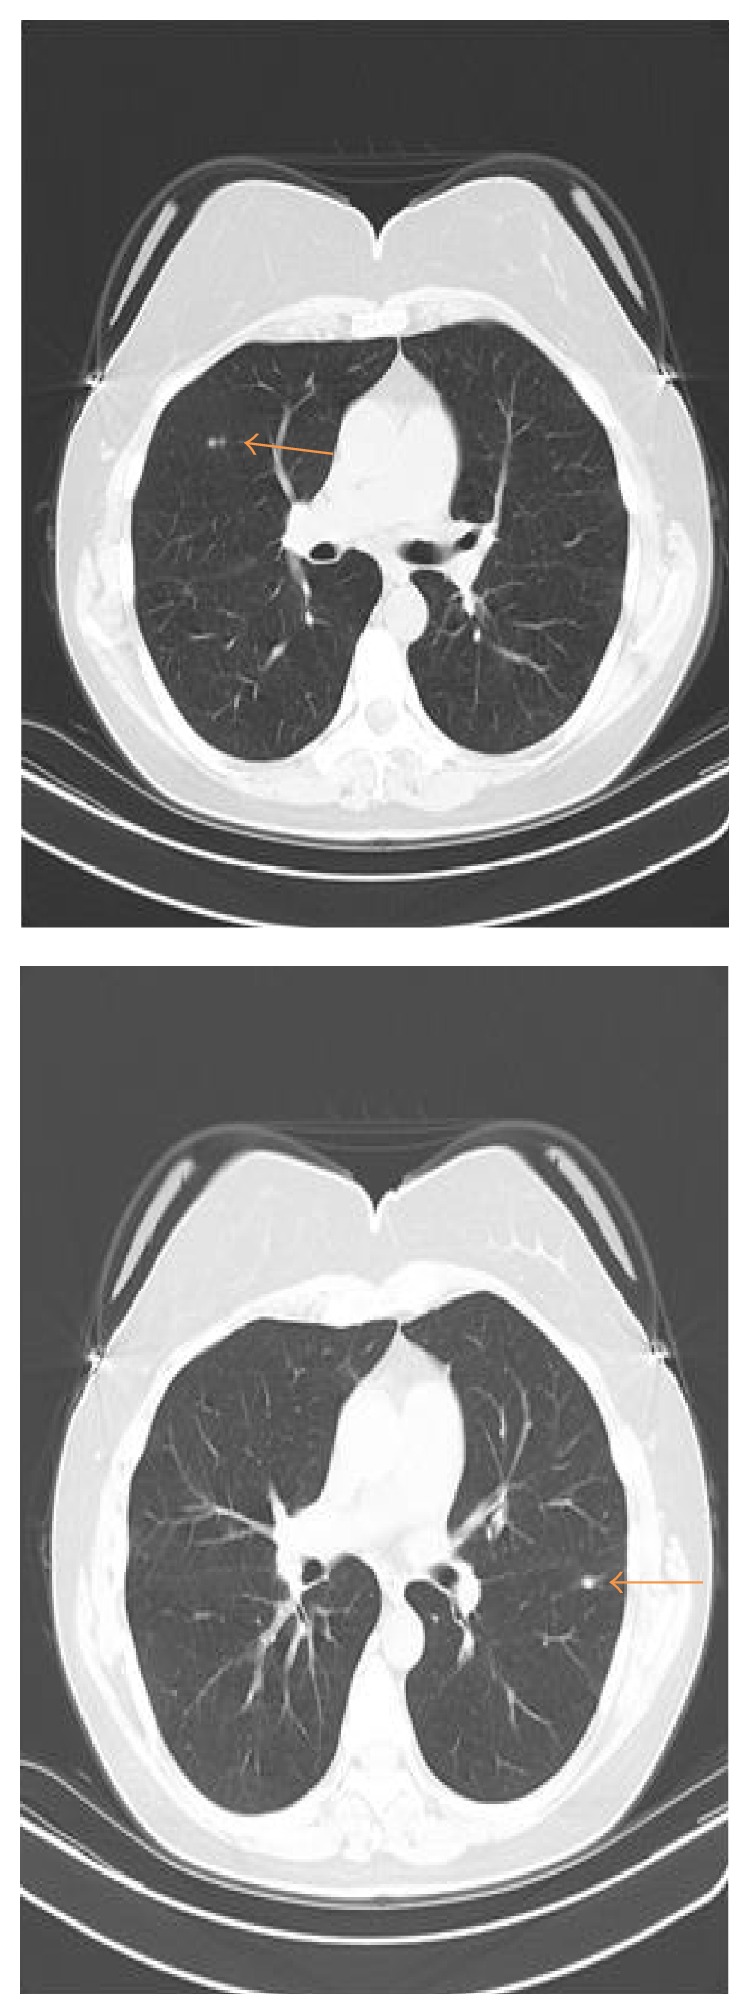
Chest CT scan, revealing bilateral nodules.
